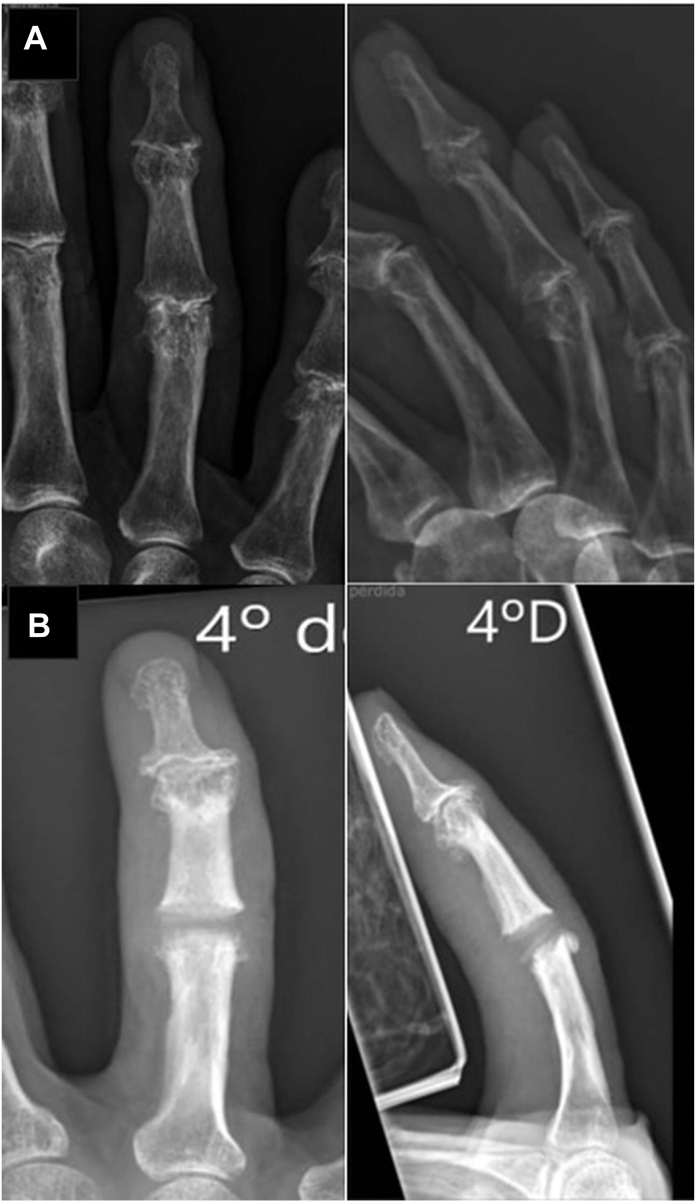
Radiographic assessment. A 74-year-old woman’s before surgery A and 30-month postoperative images B after volar approach proximal interphalangeal joint (PIPJ) arthroplasty.
